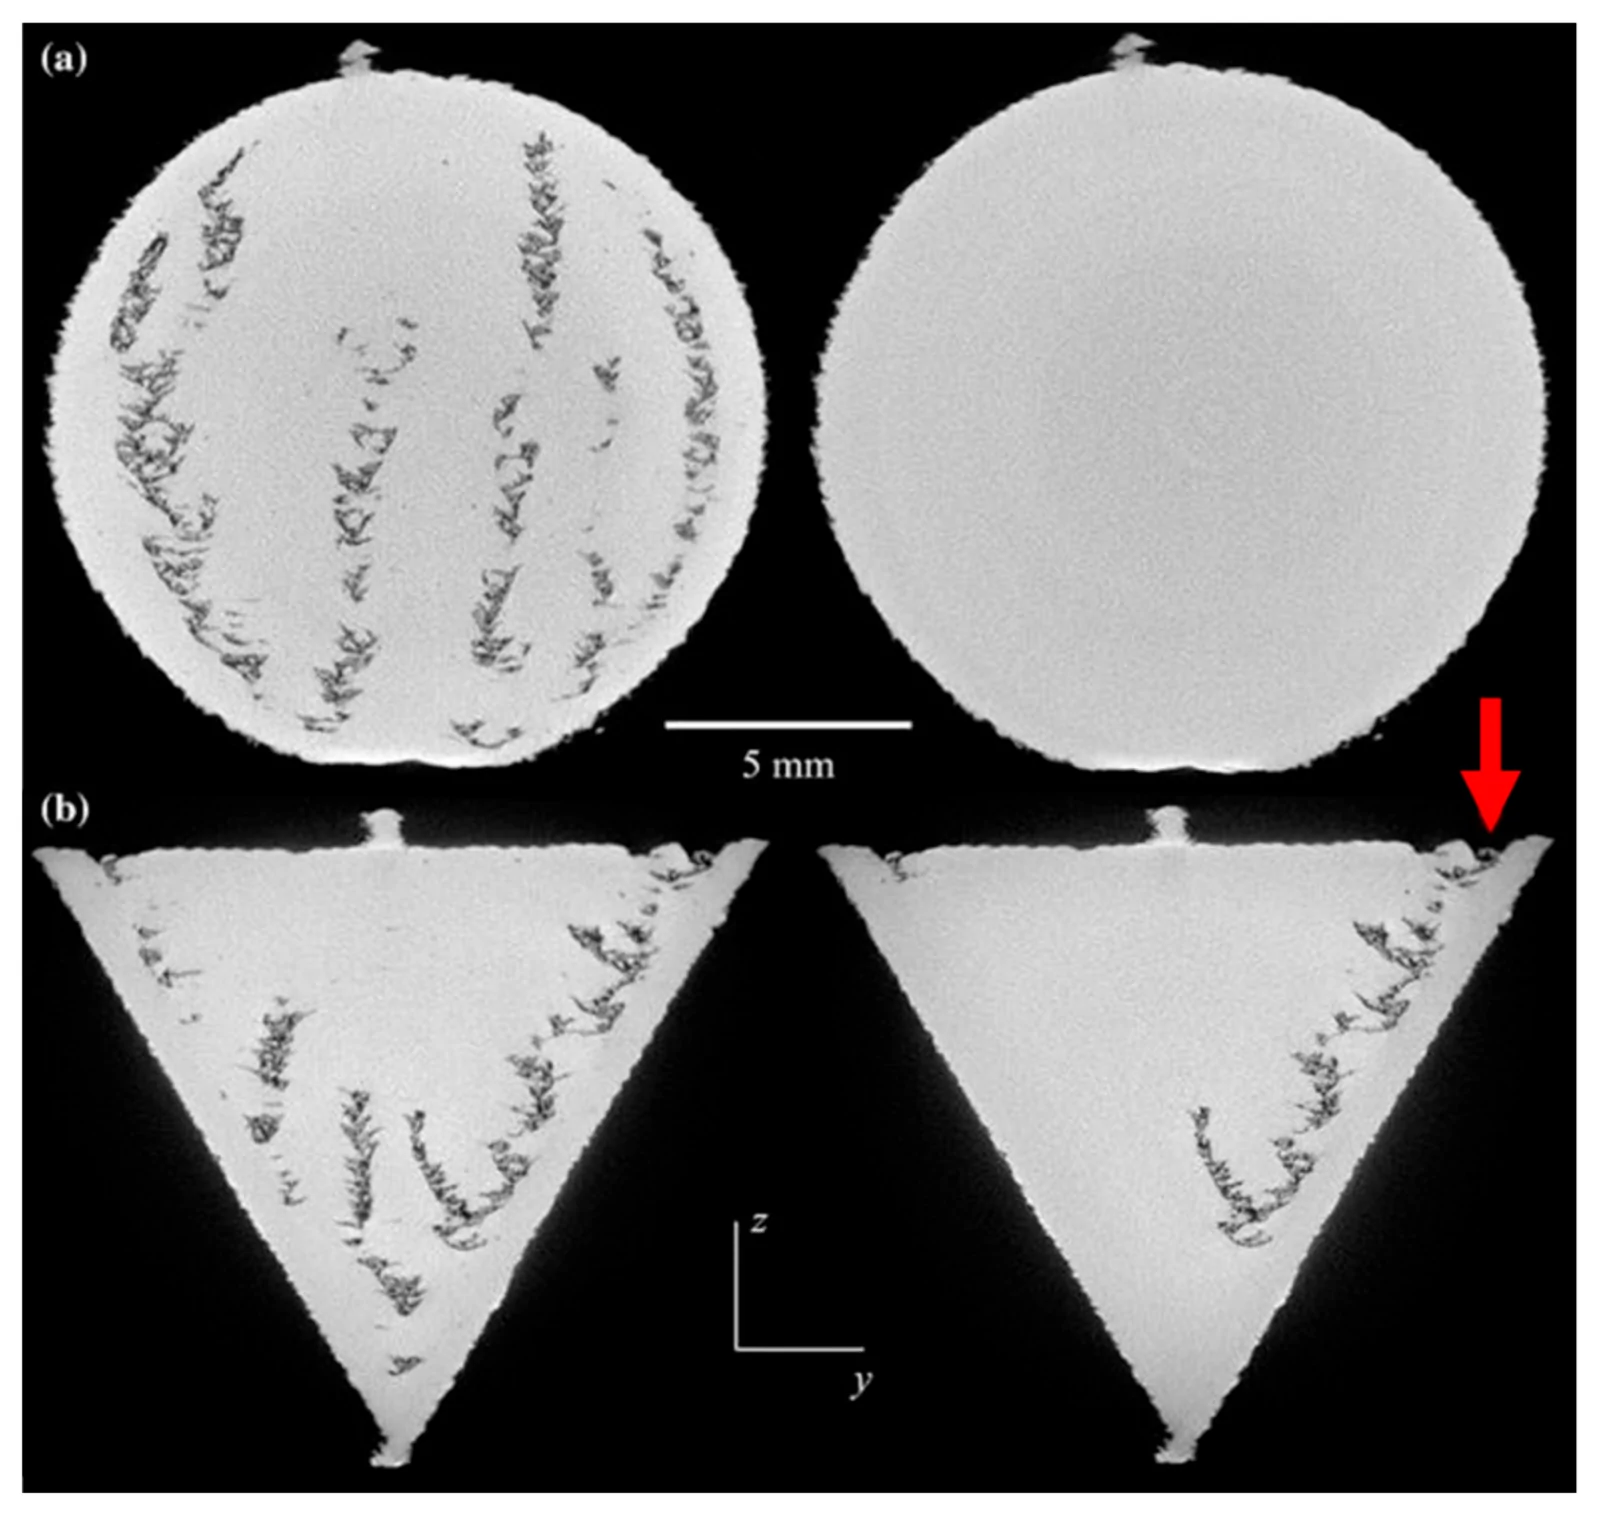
Slices of PBF Ti-6Al-4V from X-ray computed tomography data collected as-built (left) and after HIPing (right) in (a) cylindrical and (b) triangular prism samples. Red arrow in (b) shows a tunnel-like surface-connected defect present after HIPing. The build direction for both samples is along *z*-axis [20]—reproduced with modifications under Creative Commons (CC BY) license.
